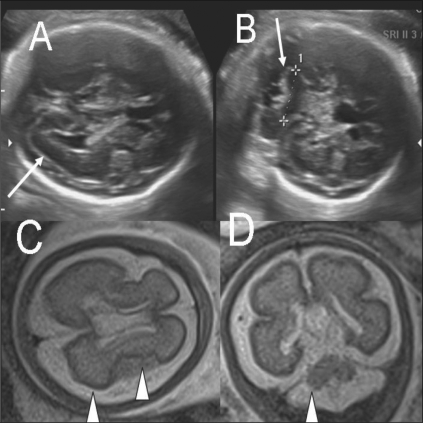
Single live gestation at 31 weeks. USG images (A,B) show suspected cortical atrophy (arrow in A) and cerebellar hypoplasia (arrow in B). Axial T2W HASTE MRI image (C) shows poor sulcation and shallow Sylvian fissures with poor opercularization (arrowheads), diagnostic of lissencephaly. Coronal T2W MRI image (D) shows cerebellar hypoplasia (arrowhead). This case was lost to follow-up
